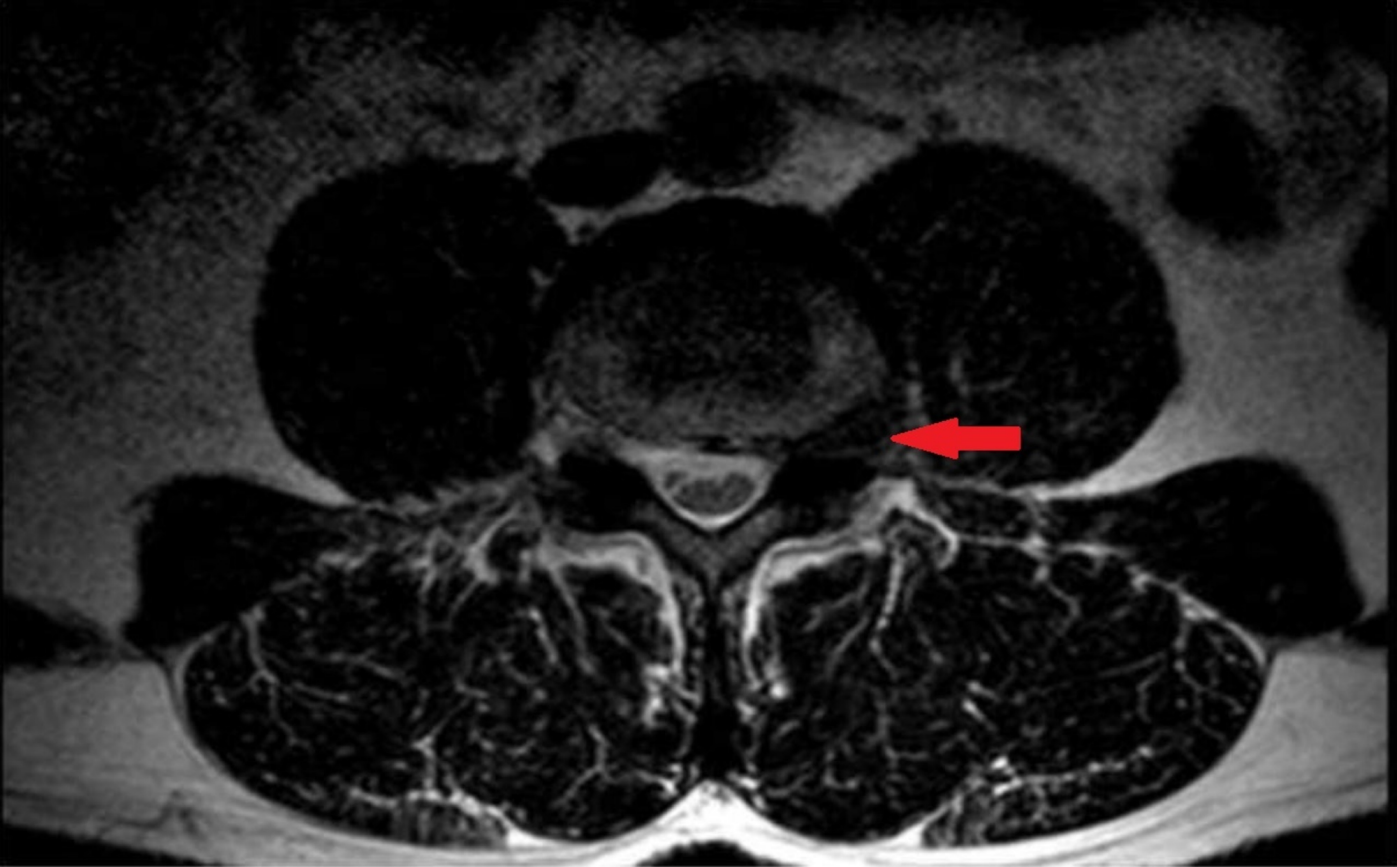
Axial non-contrast T2-weighted magnetic resonance image demonstrating a left far-lateral disc herniation at L3-4 (red arrow).
